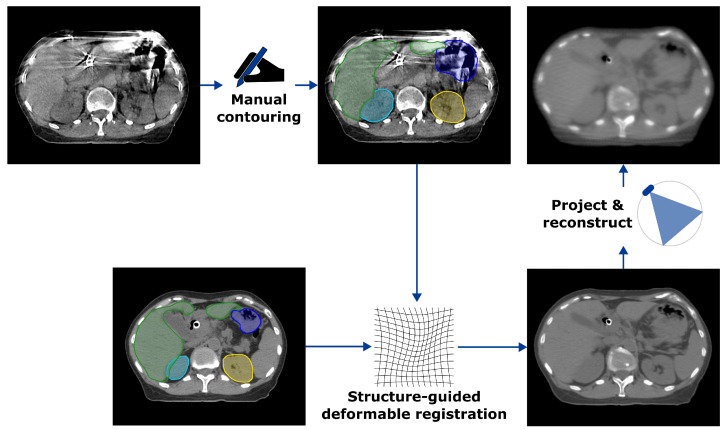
Synthetic CBCT generation process. Contours of the stomach (blue), liver (green), and both kidneys (cyan, yellow) manually drawn on daily CBCTs are used to drive a structure-guided deformable image registration from the planning CT with clinical contours to the daily CBCT. Analytical projections are then acquired of the deformed CT and reconstructed to produce the synthetic CBCT data.
